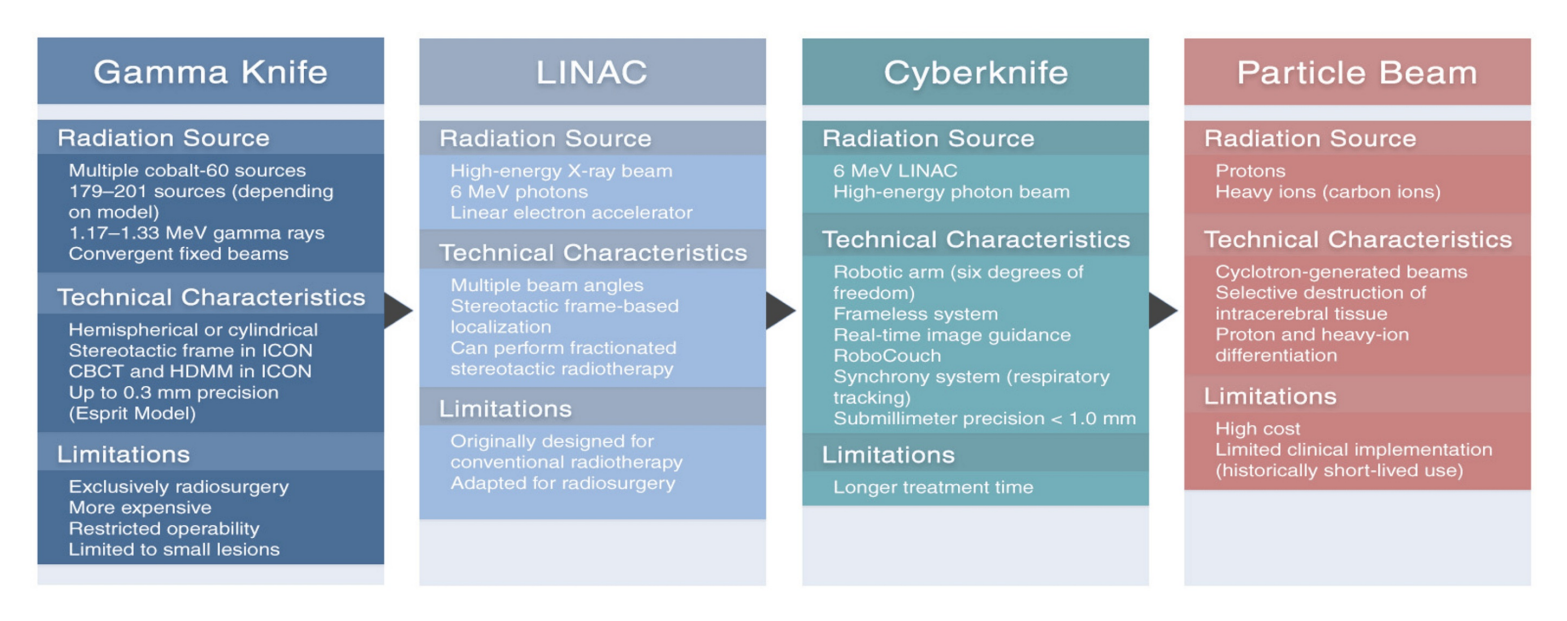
Radiosurgery technologies; Gamma Knife, LINAC, CyberKnife and particle beam compared. Figure created by the authors using Adobe Photoshop 2023 (Adobe Inc., San Jose, CA, USA). LINAC, linear accelerator; CBCT: cone-beam computed tomography; HDMM, high-definition motion management during fractionation (HDMM).
